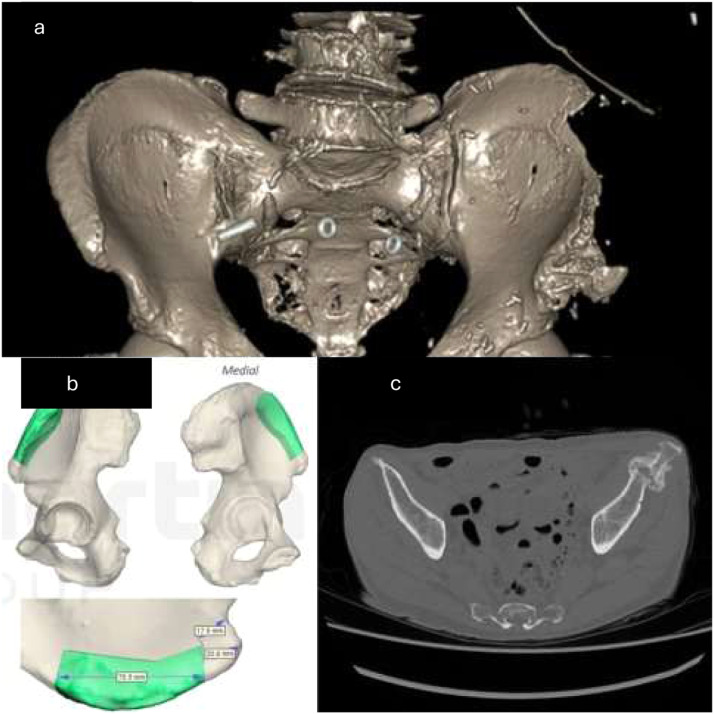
A CT imaging demonstrated an anterosuperior iliac crest fracture (Type A). Fig. 2B. Surgical planning analysis showed that 75.9 mm of iliac crest bone had been harvested, with 20.6 mm distance from the ASIS to the osteotomy site. Fig. 2C. Axial view of the CT scan showing callus formation within the fracture. The patient was managed conservatively following orthopaedic consultation, with physiotherapy and gradual mobilization. He achieved good functional recovery.
